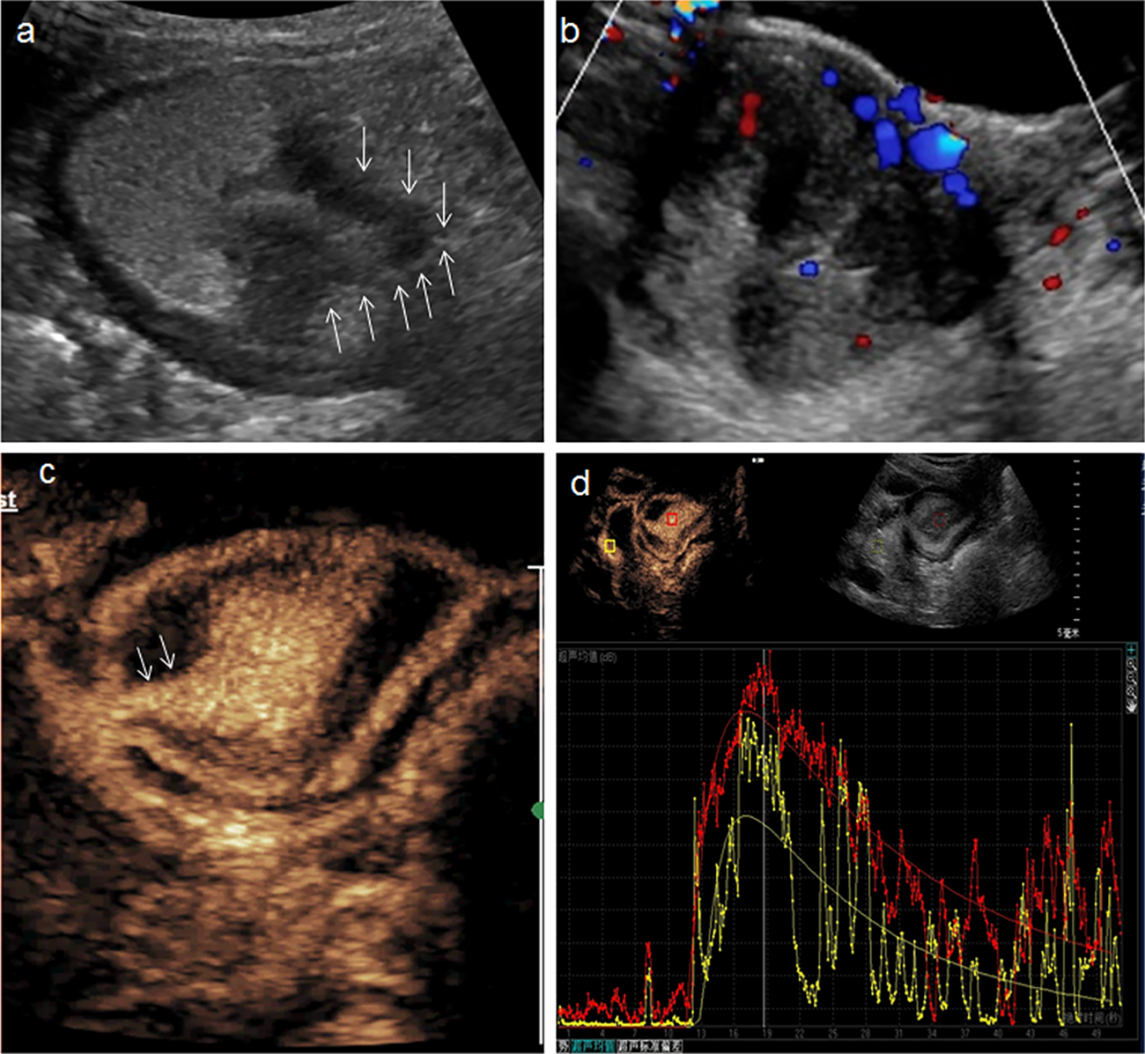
A 65-year-old man with a polypoid adenoma. a: Conventional trans-abdominal ultrasound with oral contrast agent showed an irregular hypoechoic lesion (arrow) located in antrum. b: Multiple flow signals were visible on the color Doppler. c: DCUS showed homogeneous hyper-enhanced mass connected with gastric wall by a pedicle (arrow). d: Time-intensity curve depicted the lesion (red line) have higher PI than surrounding normal tissue (yellow).
